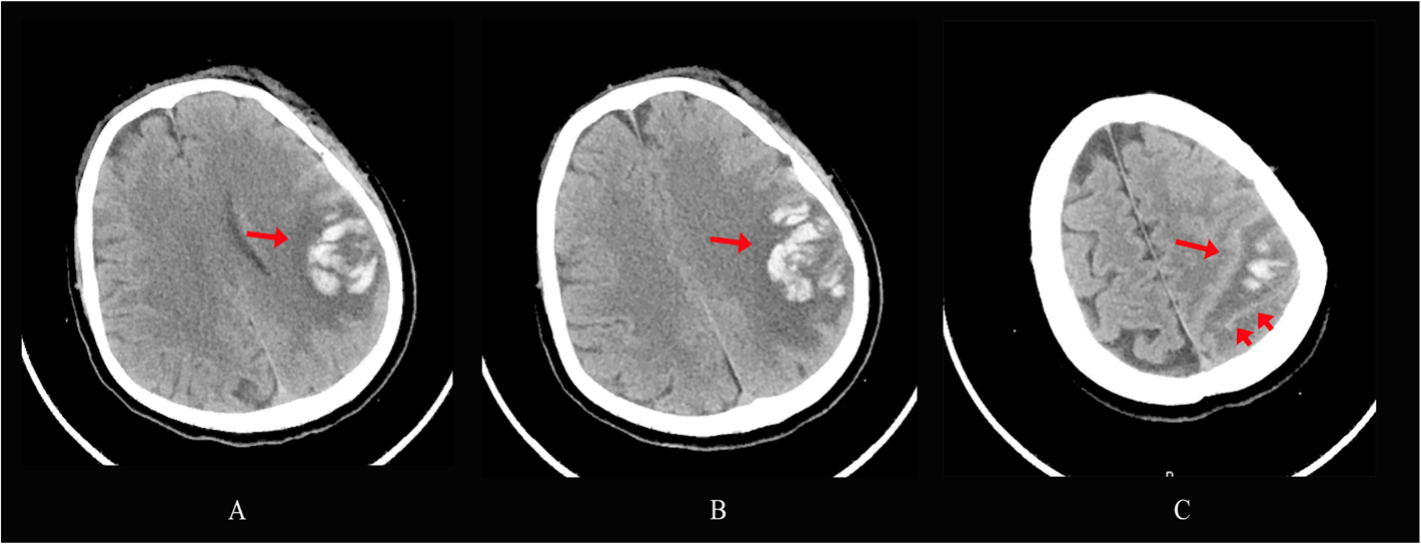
Head CT upon admission showed hemorrhagic infarction mainly involving the left parietal lobe (a-c, arrow) and subarachnoid hemorrhage (c, arrow heads)
